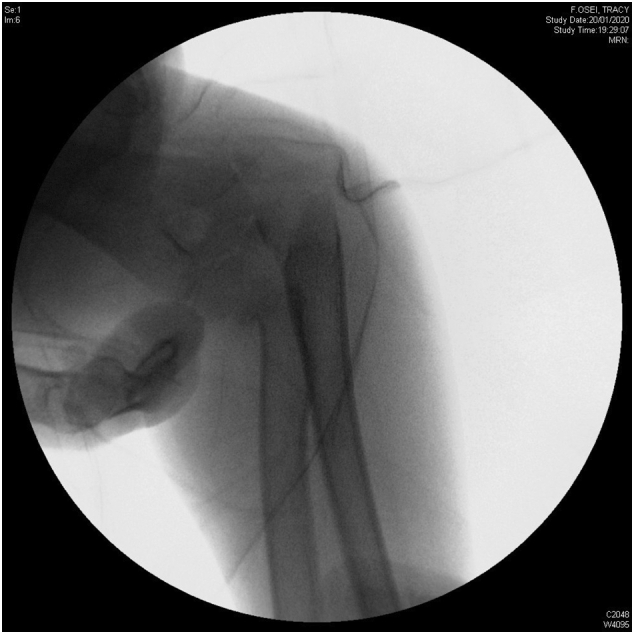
Stress radiographs performed in the operating room revealed an associated severe valgus instability caused by medial collateral ligament disruption.
